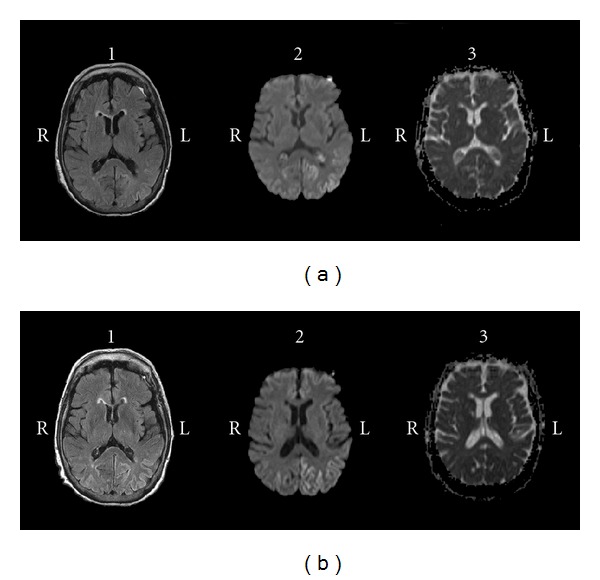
(a) Fluid attenuated inversion recovery (FLAIR), diffusion weighted imaging (DWI) and apparent diffusion coefficient (ADC) MRI demonstrate bilateral temporal-occipital cortex predominantly left hemispheric alterations with integrity of basal ganglia. (b) MRI images obtained two months later revealing the progression of the abnormalities.
